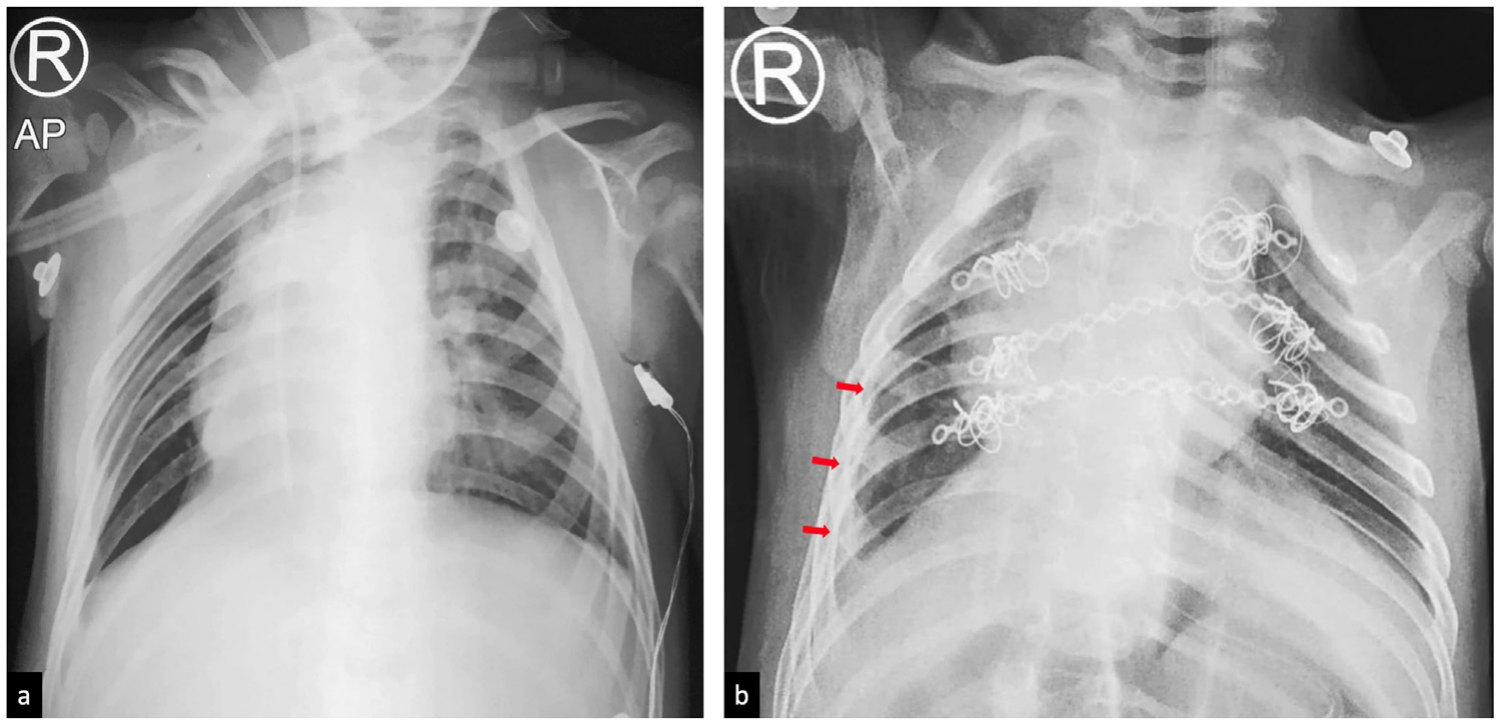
Post-surgery X-ray evaluations. (a) Four days after surgery, AP projection chest X-ray (rotated) of the right baby appears normal; (b) nineteen days after surgery, AP projection chest X-ray of the left baby shows dextrocardia (heart apex at right side) and laminar pleural effusion (red arrow). The left baby did not have a sternum and temporary internal fixation was placed to allow the lung to expand properly; when she is older, it will be replaced with a proper implant (Color version of the figure is available online.)
